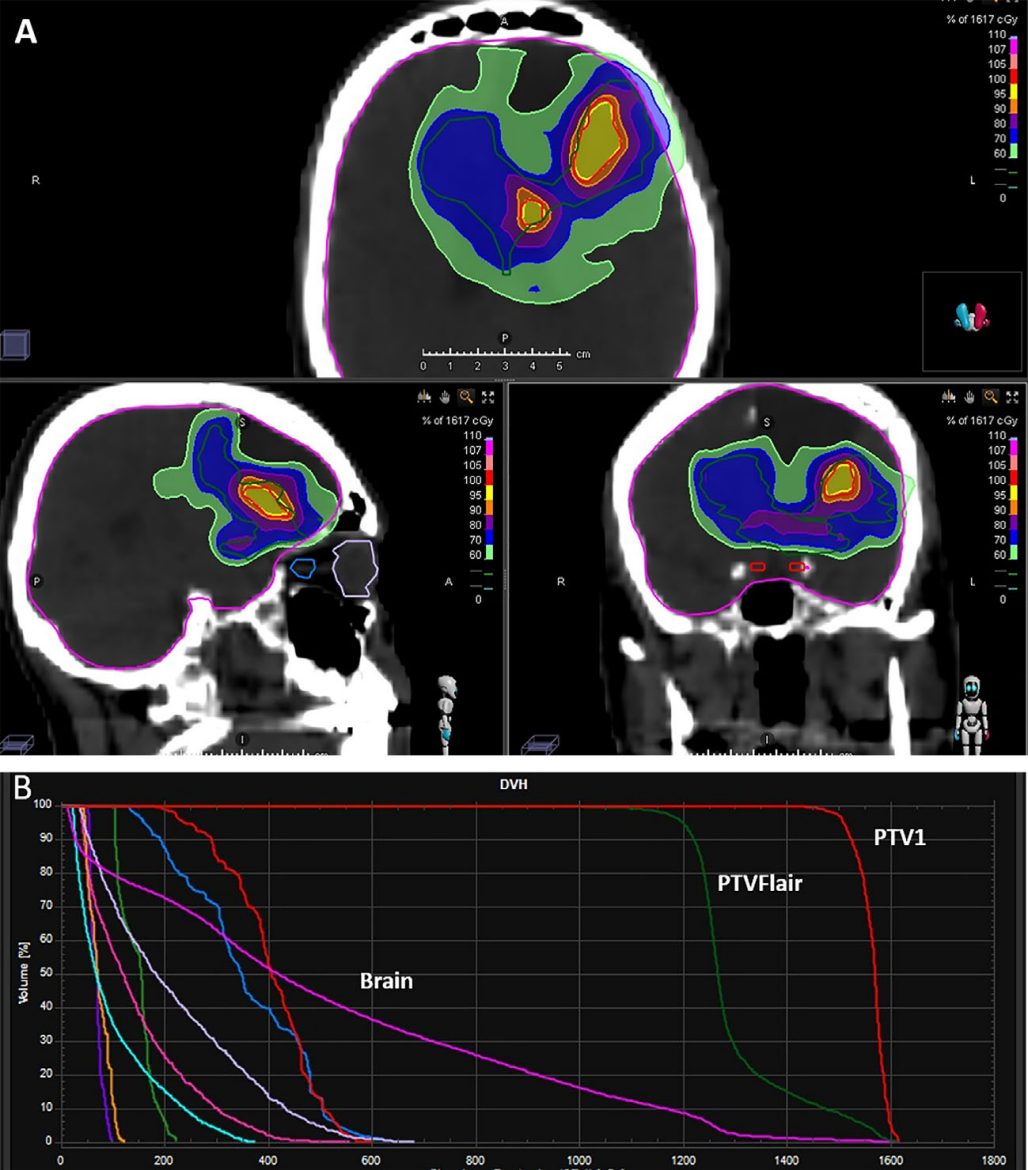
Examples of (A) dose distribution and (B) typical dose volume histogram (DVH) for a prescription dose of 15 Gy in 3 fractions to PTV1 and 12 Gy in 3 fractions to PTV FLAIR.
